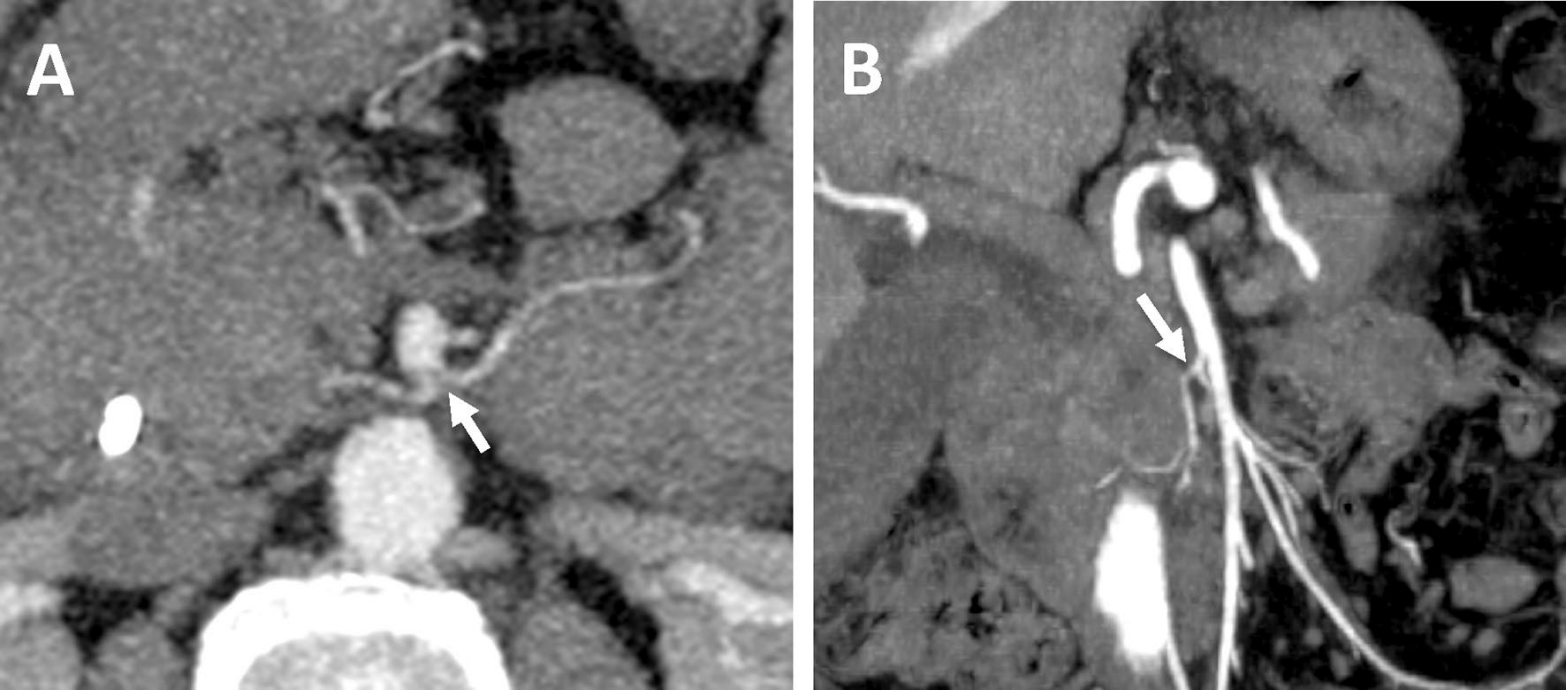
CT-examinations of two different patients with adenocarcinoma of pancreatic head obtained with intravenous contrast material. (A) Axial MIP-image in arterial phase shows the common origin of inferior posterior pancreatoduodenal artery and first jejunal branch (arrow), arising from superior mesenteric artery (B) oblique coronal MIP-image shows inferior pancreatoduodenal artery and its branches with its common origin with the first jejunal branch (arrow).
